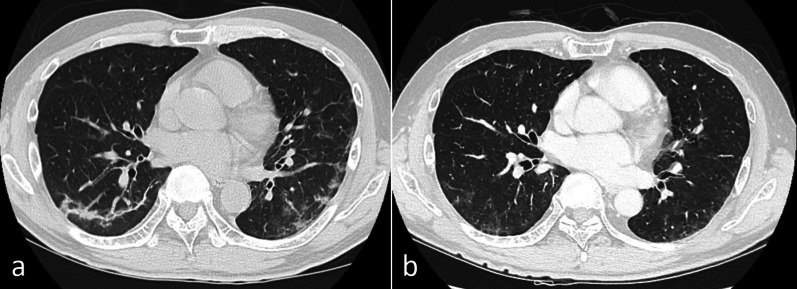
Findings of case 2 with chest computed tomography. a A crazy-paving pattern and small ground-glass opacities were located peripherally in bilateral lower lobes immediately after COVID-19 infection. b Ground-glass opacities were still present 19 days before the operation
